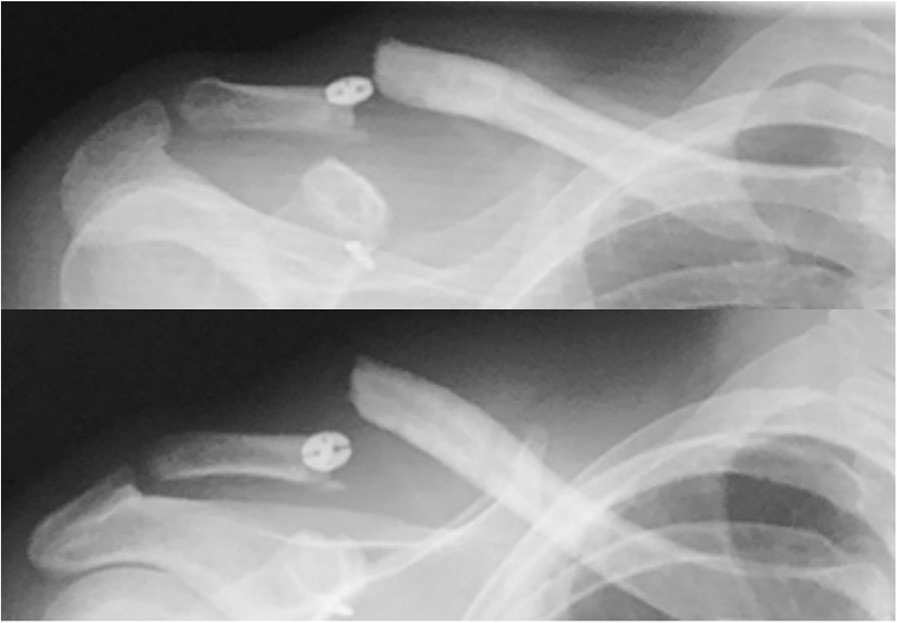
X-ray radiography images at the second injury revealed a clavicle fracture at the suture hole
